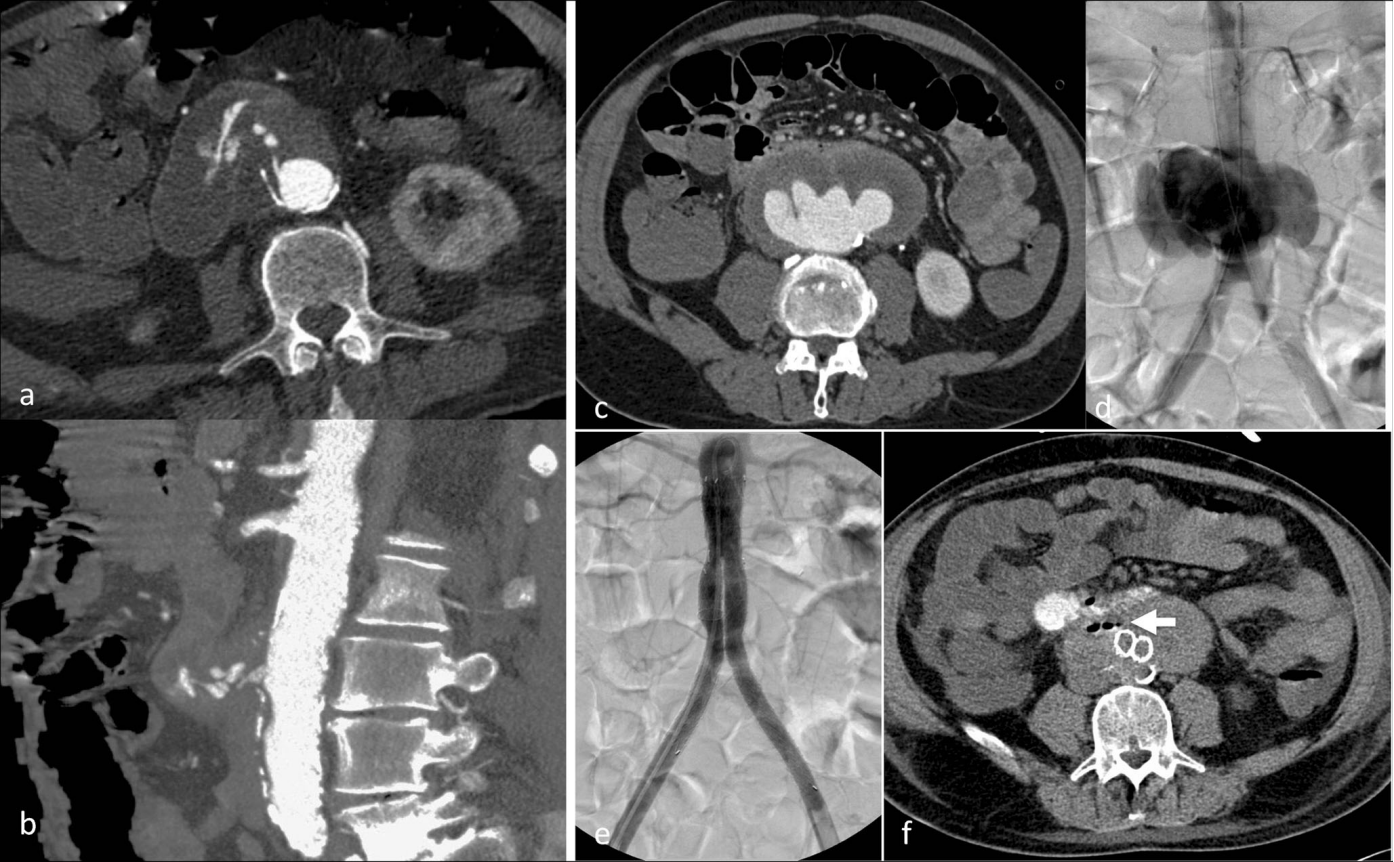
Aorto-duodenal fistulae in a 75-year-old male patient presenting with melena and haematemesis. He had an aortic repair with a surgical tube graft 10 years ago. CTA (axial and coronal reformations) demonstrates contrast extravasation of the infrarenal aorta directly into the adjacent duodenum (a–b). The patient died immediately after the CT scan before stent graft implantation could be performed. In a 63-year-old male patient with severe haematemesis ultrasound detected an aortic aneurysm. He had previous aortic repair (15 years ago) and had also been suffering from intermittent haematemesis for 3 months. CTA (c) and DSA (d) demonstrated an aneurysm of the distal anastomosis in close proximity to the duodenum. A bifurcated stent graft was implanted (e). The aneurysm was completely excluded. A follow-up CT on the next day (after oral contrast) nicely demonstrated the aorto-duodenal fistulae (*arrow*) (f)
